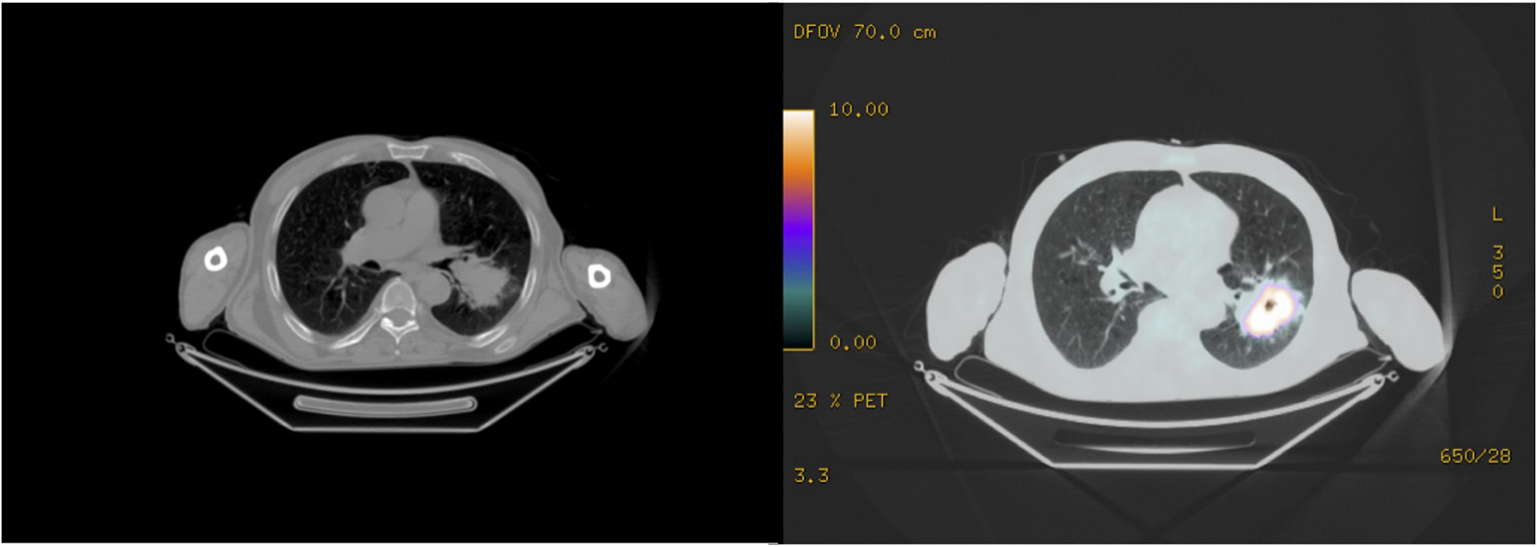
A PET-CT scan revealing the presence of a 7-cm left lung neoformation with no nodal involvement.
